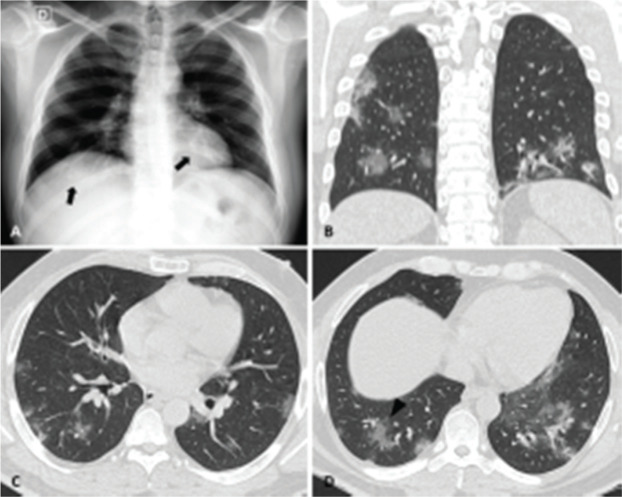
Chest radiography (A), posteroanterior view, shows retrocardiac and right peri diaphragmatic opacities (arrow), regions which are often neglected in the radiographic evaluation; there are no other notable parenchymal changes. Coronal (B) and axial (C and D) CT images on the same day show ground glass opacities associated with some consolidations in the posterior regions of both lungs, most prominent in the lower lobes. Note the slight thickening of the inter and intralobular septa that constitute the crazy-paving pattern in the right lung base (arrowhead).
